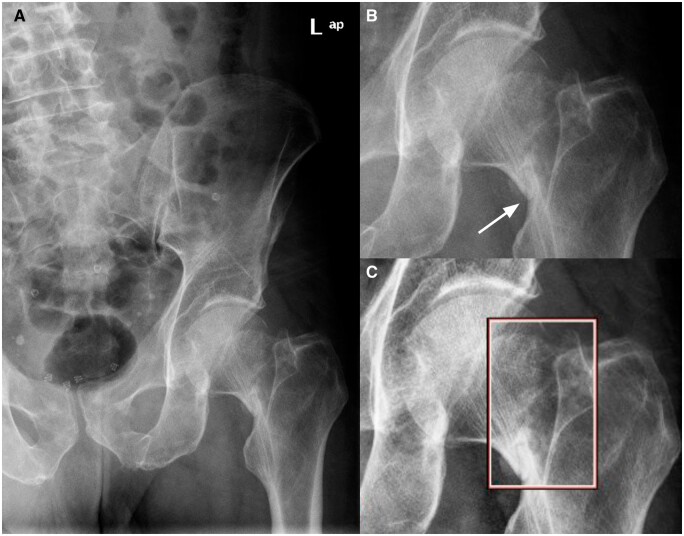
Anterior-posterior radiograph (A) of a nonobvious hip fracture unaided. The subcapital hip fracture (B, white arrow) was missed by 9 readers in the unaided exam, but detected by all readers in the aided exam, with support from the AI tool (C).
